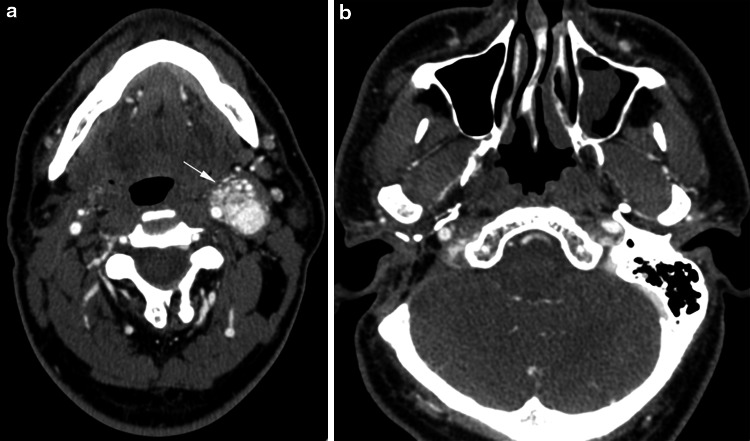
Axial post-contrast CT scans demonstrate large carotid PG (a) (*arrow*) in a patient after single-stage infratemporal removal of contralateral, synchronic jugular and vagal PGs (b)
